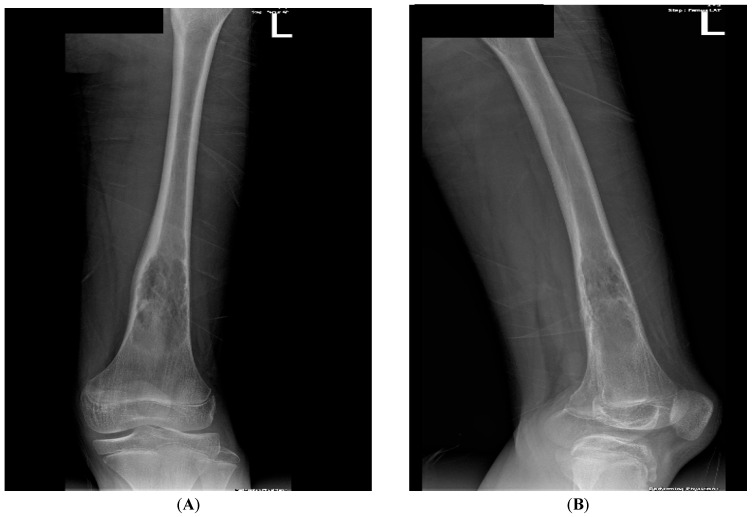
(A) Anteroposterior radiograph of the left femur showing an aggressive lytic lesion in the distal femoral diaphysis with cortical destruction. (B) Lateral radiograph confirming the extent of the diaphyseal lesion with aggressive radiographic features.
